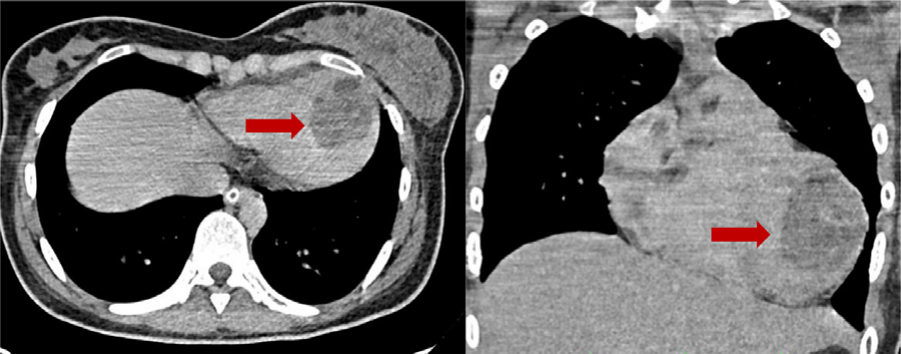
Thoracic CT scan, in axial and coronal reconstructions, showing well capsulated hypodense lesion seen in the myocardium of the left ventricle, containing multiple daughter cysts, suggestive of a hydatid cyst.
